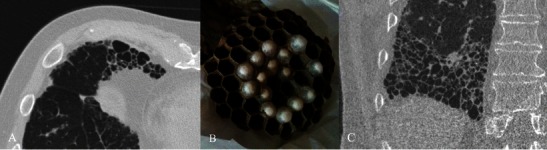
Honeycombing appearance on HRCT images. The cystic spaces reproduced on HRCT images (A and C); a real honeycomb (B, removed from apartment window of one of the authors). The honeycombing appearance consists of a cluster of cystic air spaces, usually with a diameter of 3–10 mm, although they may reach 2.5 cm in size [19, 20]
